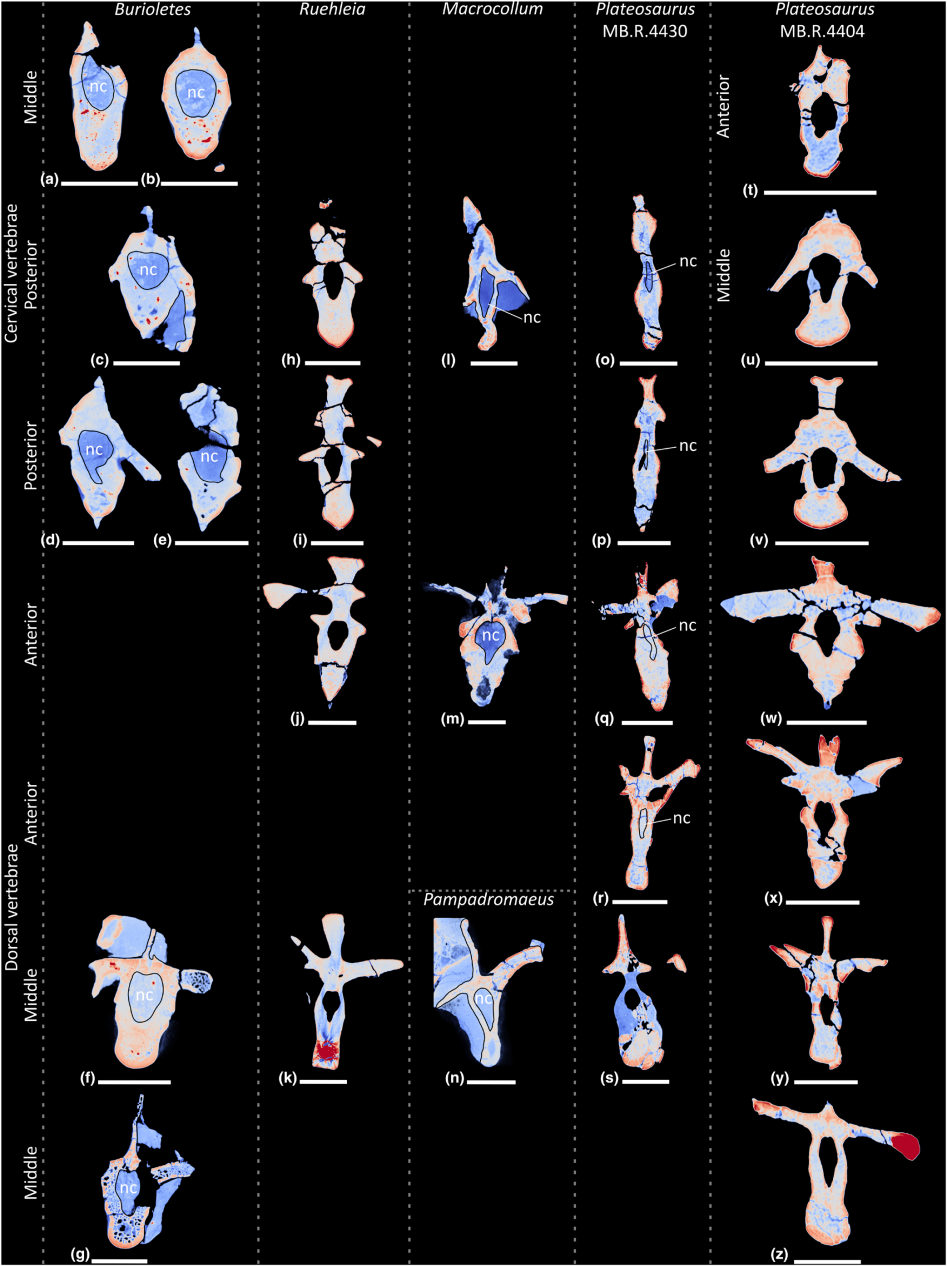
Colour density map outlining PSP in CT scan slices in transverse cross‐section of the presacral vertebrae of early‐branching sauropodomorphs. (a–g) *Buriolestes schultzi*, (h–k) *Ruehleia bedheimensis*, (l–m) *Macrocollum itaquii*, (n) *Pampadromaeus barberenai*, (o–z) *Plateosaurus longiceps*; (t) anterior cervical vertebra, (a–b, u) middle cervical vertebrae, (c–e, h–i, l, o–p, v) posterior cervical vertebrae, (j, m, q–r, w–x) anterior dorsal vertebrae, (f–g, k, n, s, y–z) middle dorsal vertebrae. nc, neural canal. Scale bars = 2 cm (*Buriolestes*, *Macrocollum*, *Pampadromaeus*), 5 cm (*Ruehleia*, *Plateosaurus*).
